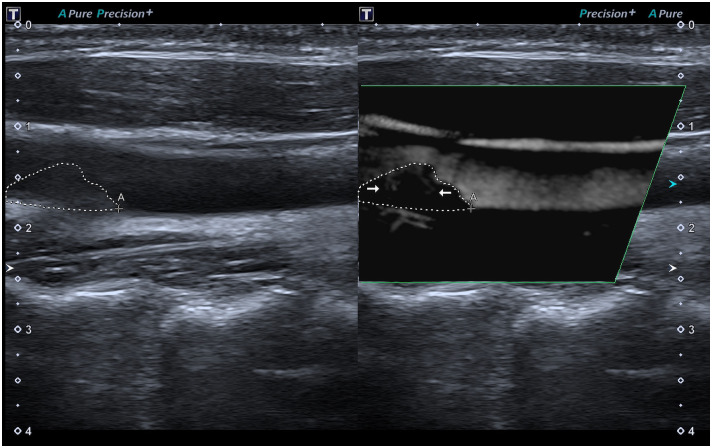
Representative SMI image of intraplaque neovascularization. B-mode ultrasound image (left) and corresponding SMI image (right) of a carotid plaque. The plaque is outlined by the dotted line, and arrows indicates microvascular signal consistent with intraplaque neovascularization (IPN).
